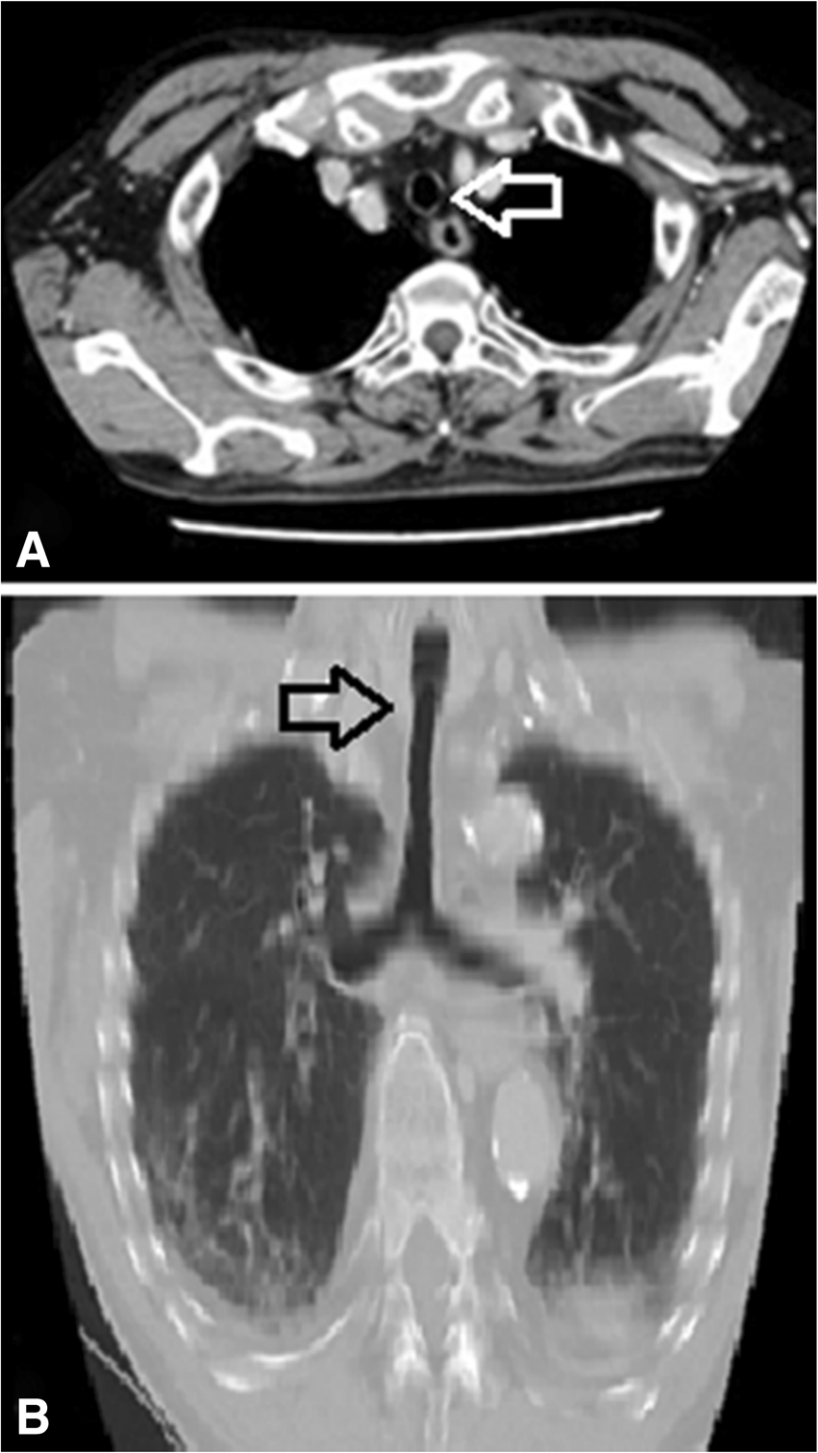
CT imaging of complete tracheal rings. a Preoperative axial CT imaging of tracheal rings (arrow). b Postoperative coronal CT reconstruction (arrow). The tracheal diameter at the site of transition to complete tracheal rings was slightly smaller than at the cricoid cartilage level. However, there was no significant stenosis
